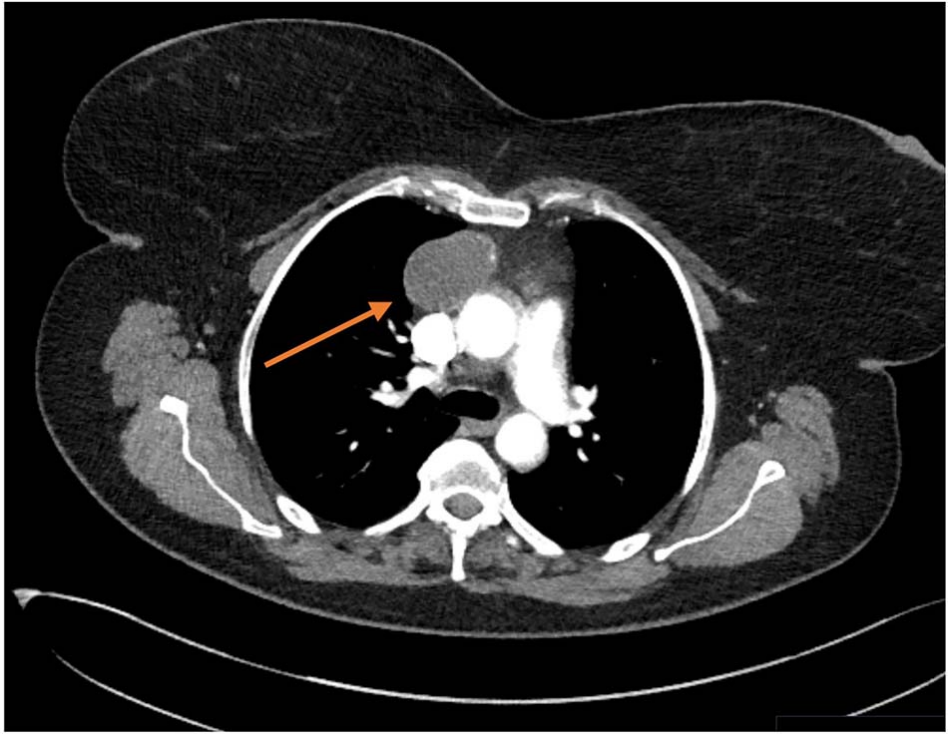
Transverse CT thorax showing anterior BMC (arrow).
